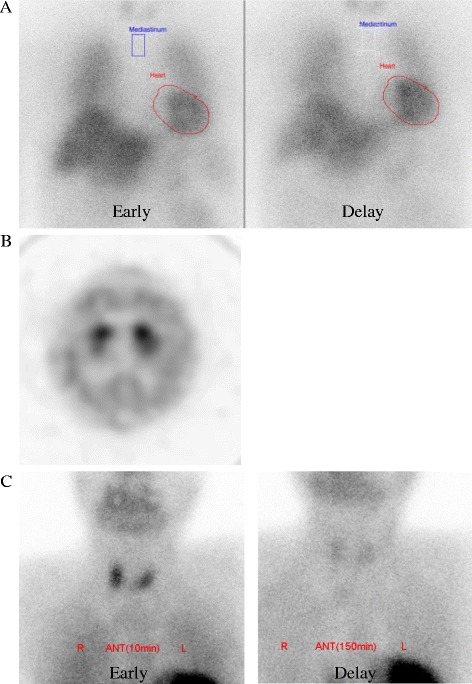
a. 123I-metaiodobenzylguanidine myocardial scintigraphy did not show a low heart/superior mediastinum ratio or acceleration of the washout rate (early and delayed heart/superior mediastinum ratio of 2.54 and 2.76, washout rate of − 5.4%). b. Dopamine transporter scan did not show a decrease in striatal accumulation. c. ^99m^Tc methoxy-isobutyl-isonitrile scintigraphy did not show abnormal accumulation in the parathyroid
